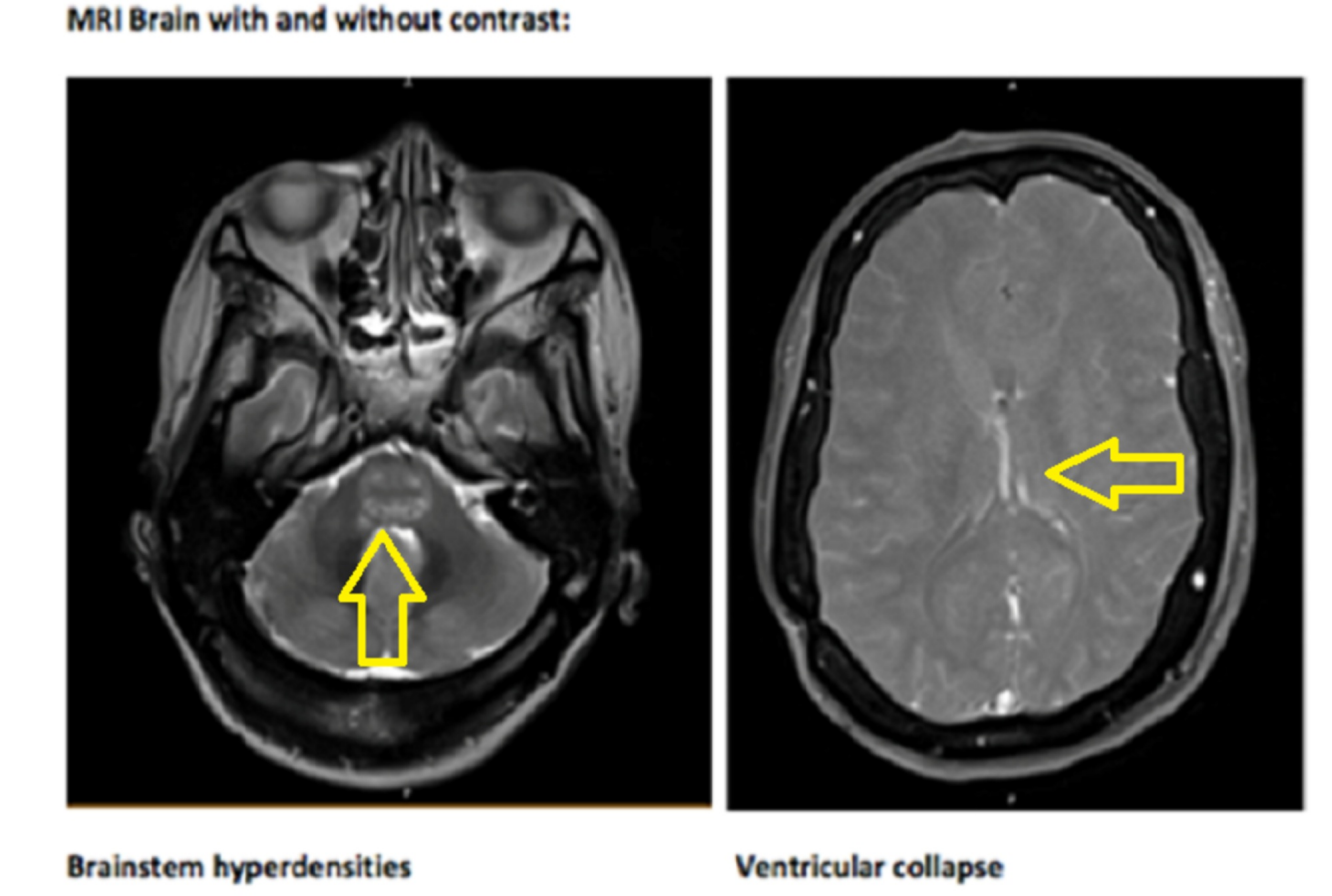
MRI Brain Showing brainstem hyperdensities and ventricular collapse
